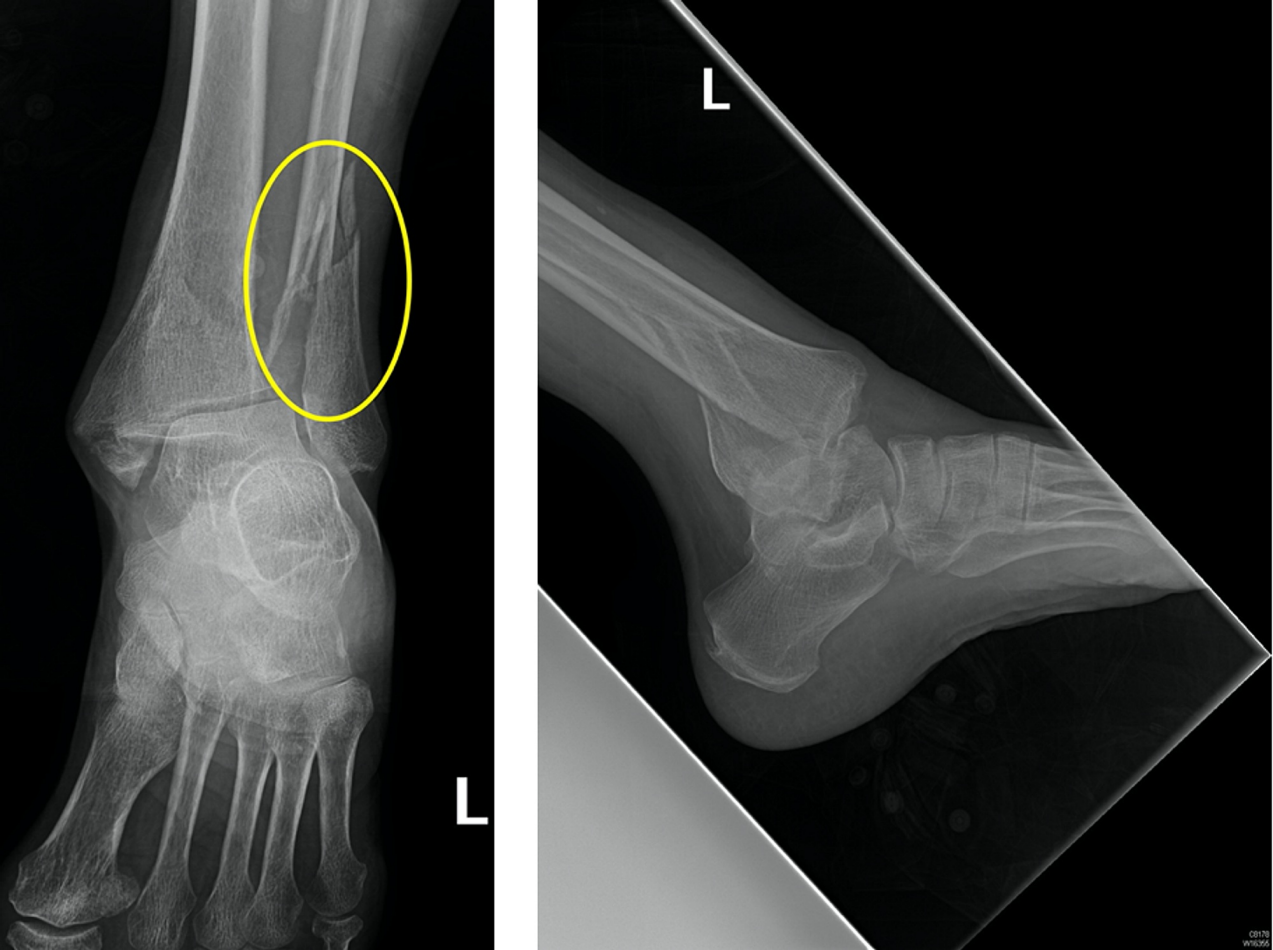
Pre-reduction anterior-posterior and lateral X-ray views demonstrating comminuted displaced trimalleolar fracture with a yellow circle highlighting the distal fibular fracture component
